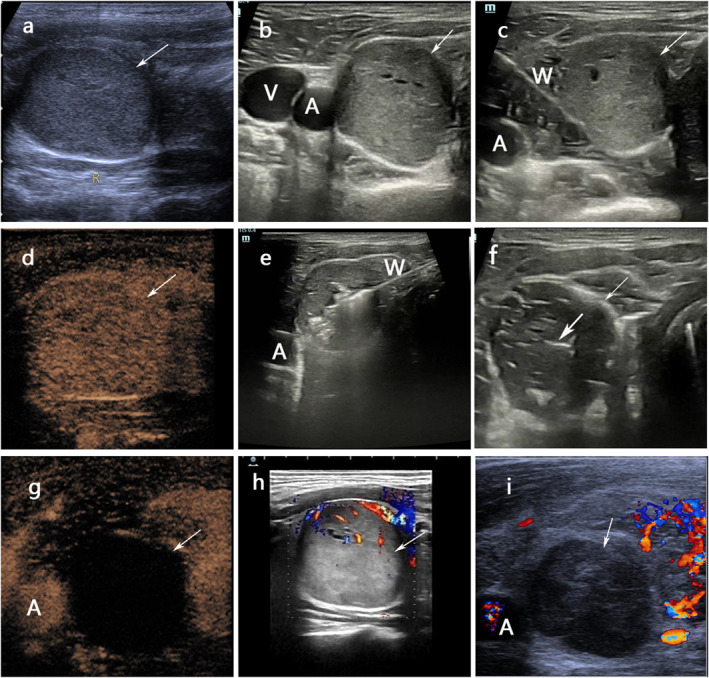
A 11‐year‐old boy received microwave ablation (MWA) for a solid benign nodule in the right lobe of his thyroid gland. (A) Longitudinal image before MWA showed a isoechoic solid nodule (thick arrow) with peripheral halo (the nodules were measured as4.0 cm × 2.44 cm × 2.03 cm). (B) Transverse images before treatment showed the nodules adjacent carotid artery(A). (C). Saline(W) is injected separately into the carotid sheath, thyroid lateral, and posterior spaces to form a water barrier to protect the recurrent laryngeal nerve and blood vessel. (D) CEUS before treatment displays hyperenhancement of the nodule. (E) Microwave antenna (M) was positioned in the nodule, “moving‐shot” technology was used until the nodule was covered by the hyperechoic microbubbles. (F) There were necrotic tissue (the thick arrow) in the nodules after MWA. (G) CEUS after treatment, no contrast agent was presented in the nodule. (H) Color Doppler showed intra‐nodular with vascularity before MWA. (I) Color Doppler shows intra‐nodular without vascularity.
